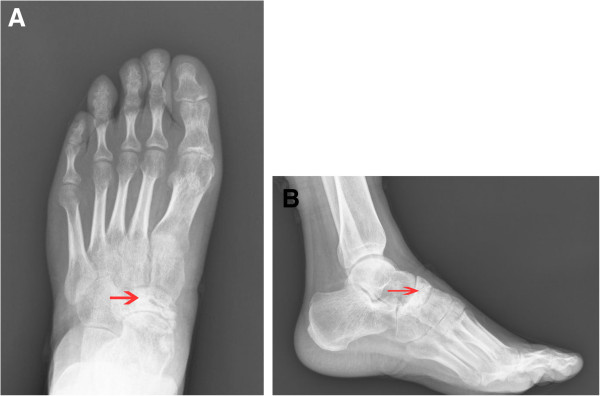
A-B Post-operative radiographs. Both anteroposterior and lateral views showed no recurrence of the tumor.
